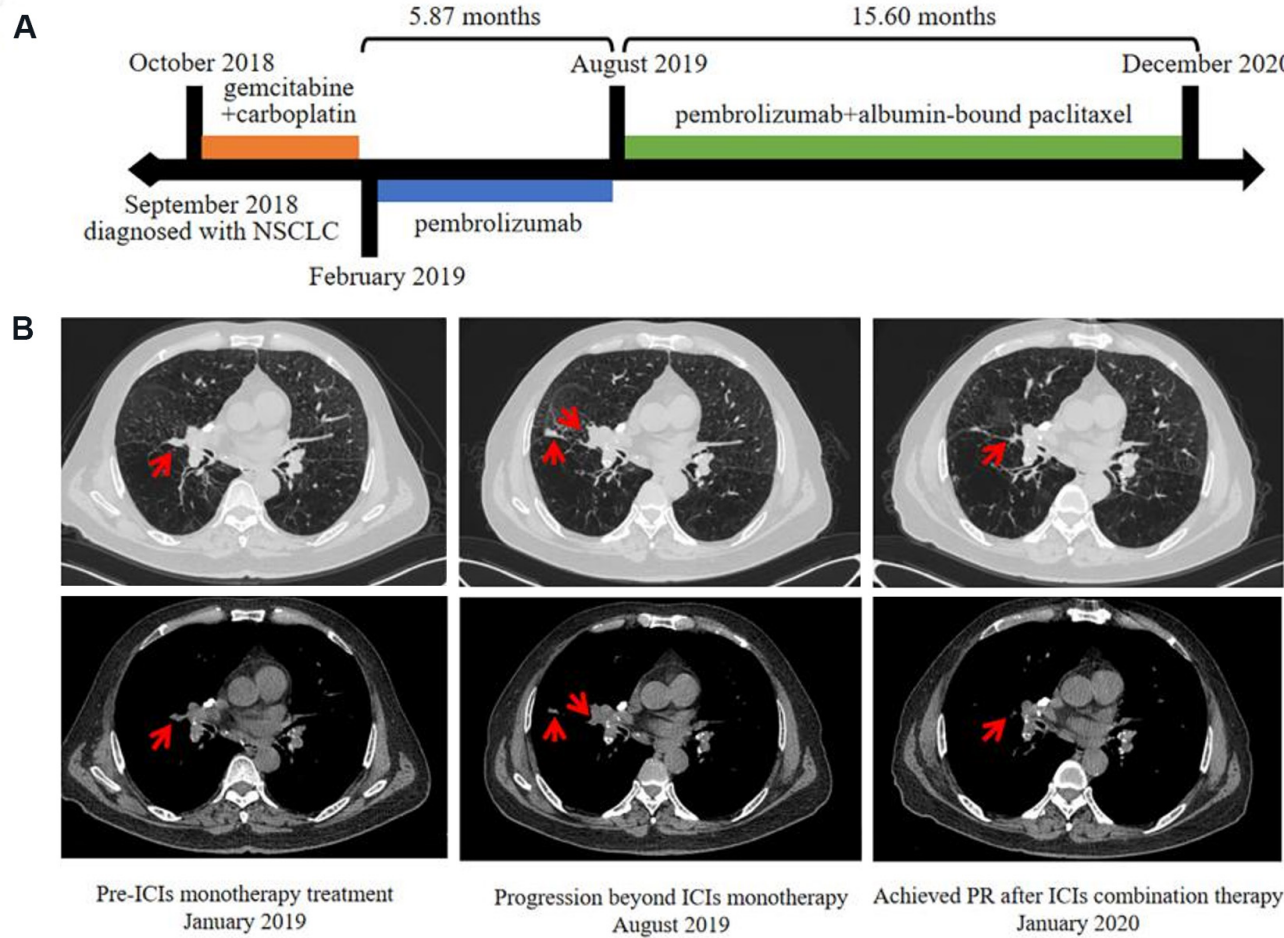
Durable clinical response to pembrolizumab plus albumin-bound paclitaxel in an NSCLC patient. (A) Clinical timeline of patient, with major treatment indicated. The patient has been benefiting from ICIs combination therapy for more than 15.60 months. (B) Chest computed tomography (CT) of the tumors before pembrolizumab initiation (January 2019), disease progression (August 2019), and achieved PR after ICIs combination therapy (October 2019). ICIs: Immune checkpoint inhibitors; NSCLC: non-small-cell lung cancer; PR: partial response.
